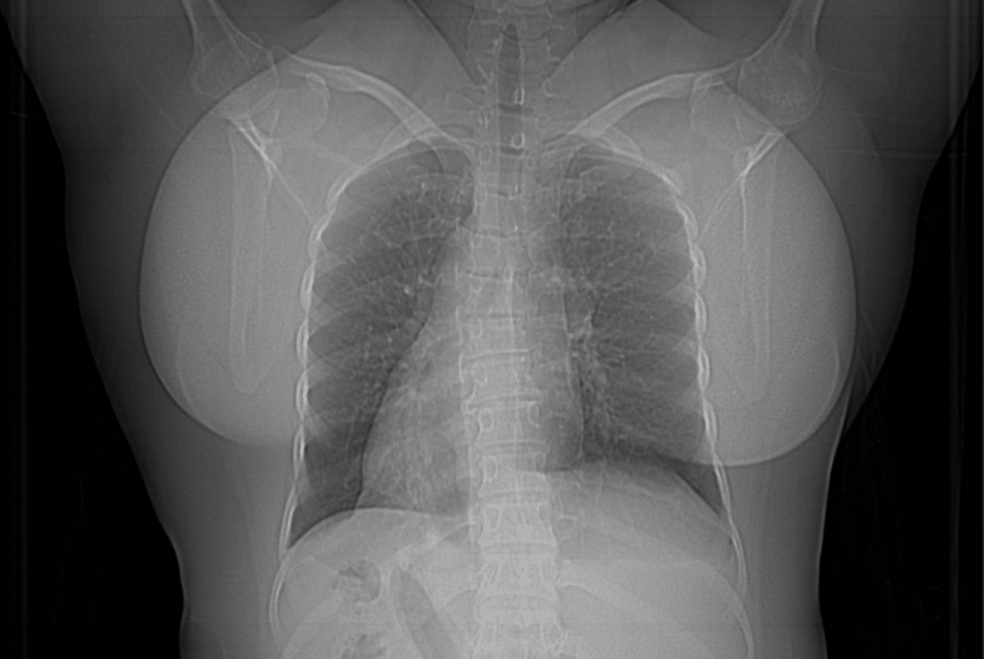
Chest X-Ray shows dextrocardia.
